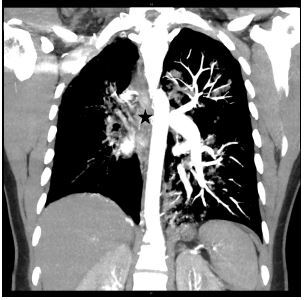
A coronal view of a contrast-enhanced computed tomography angiography revealing an absent right pulmonary artery (star) and hypoplastic right lung.
